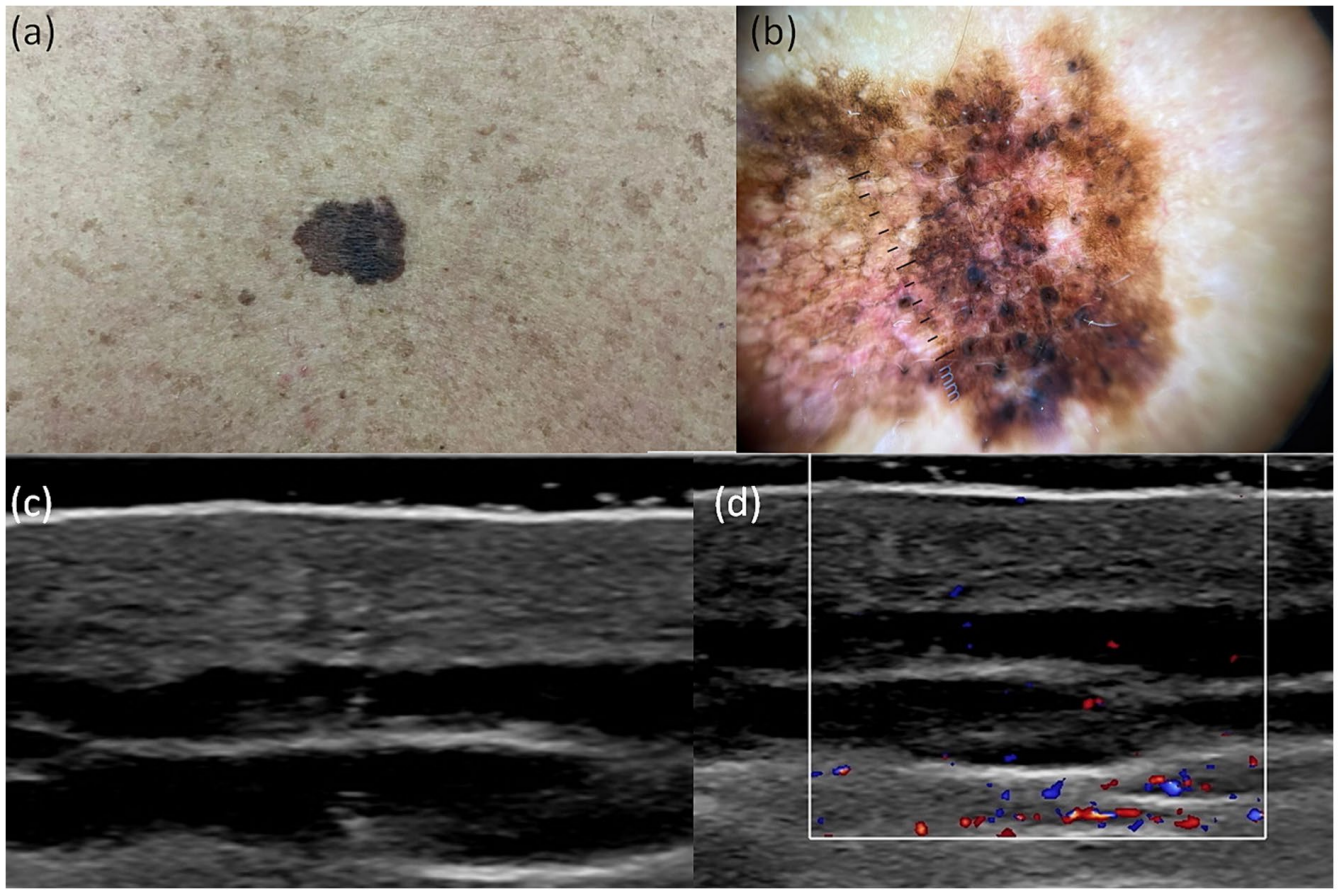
Clinical picture of MIS (A). Dermatoscopy shows the presence of an atypical network with hyperpigmented areas, angulated lines, regression structures, and irregular dots (B). US shows a hypoechoic, oval, ill-defined lesion that reaches the level of the epidermis, loss of the bilaminar pattern of the epidermis (C). Doppler mode shows no sign of regional hypervascularity (D).
